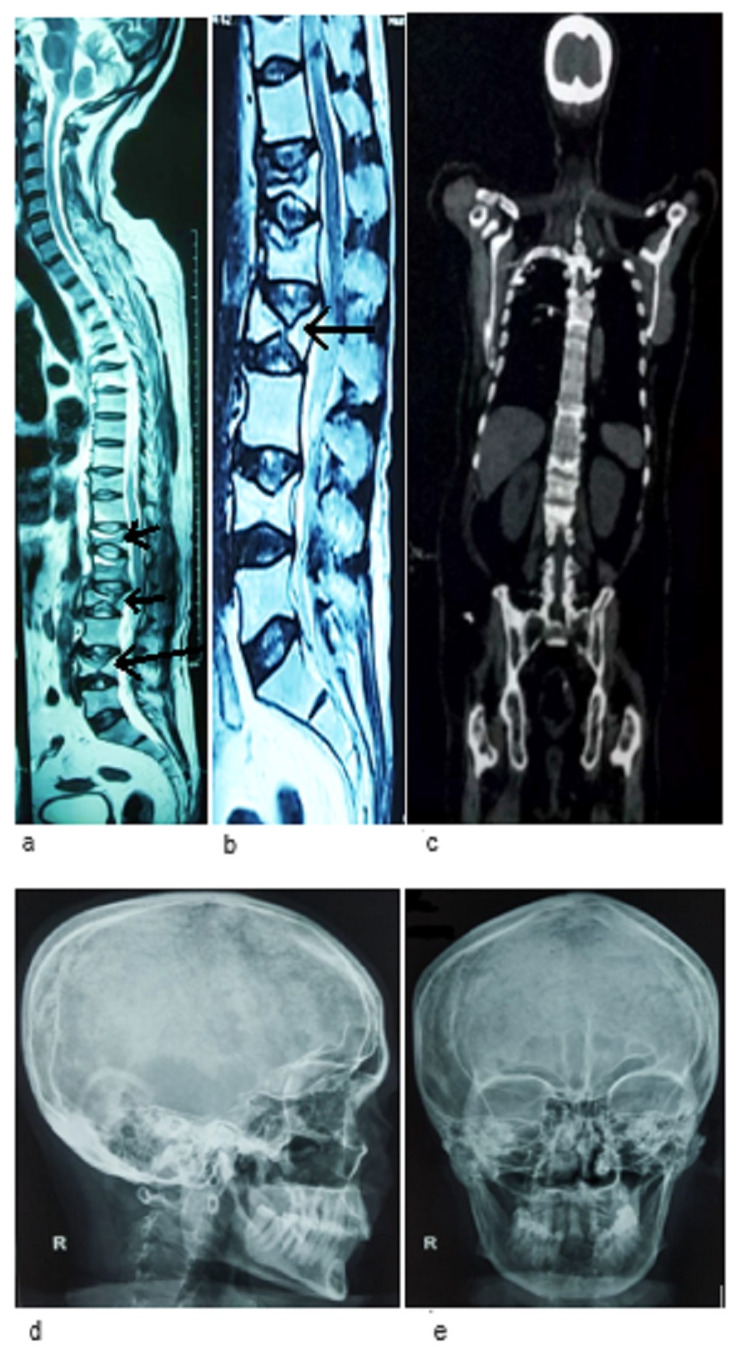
Radiographic findings of the patient. A: MRI of the dorsolumbar spine showing the partial collapse of D5, D6, D10, D12, L1, and L4 vertebrae and irregularity of the endplate with marrow edema. A diffuse disc bulge indenting the anterior thecal sac can be seen at the L1-2, L3-4, L4-5, and L5-S1 levels in association with bilateral neural foramina compression (March 2019). B: MRI of the spine revealing lumbar spondylosis with anterior wedge compression fracture of the L4, L2, L1, D12, D10 vertebral bodies (August 2019). C: PET-CT images showing few inflammatory lymph nodes suspicious for metastasis (March 2019). D, E: Lateral and frontal X-ray images of the skull showing no evidence of lytic or punched-out lesions (March 2019). PET-CT: positron emission tomography-computed tomography; MRI: magnetic resonance imaging
